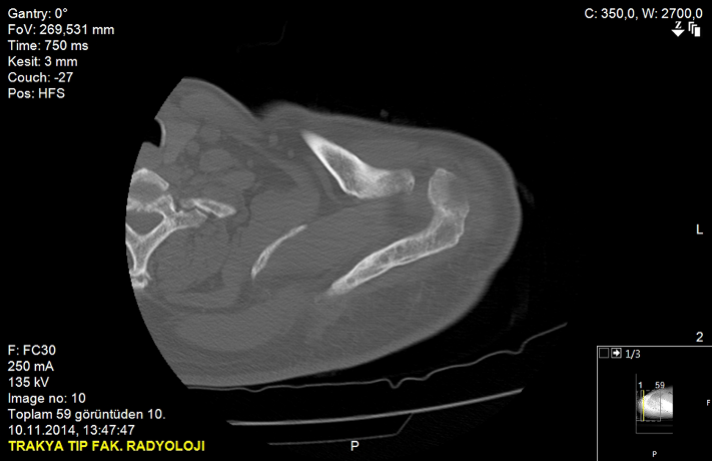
Follow-up computed tomography axial section
